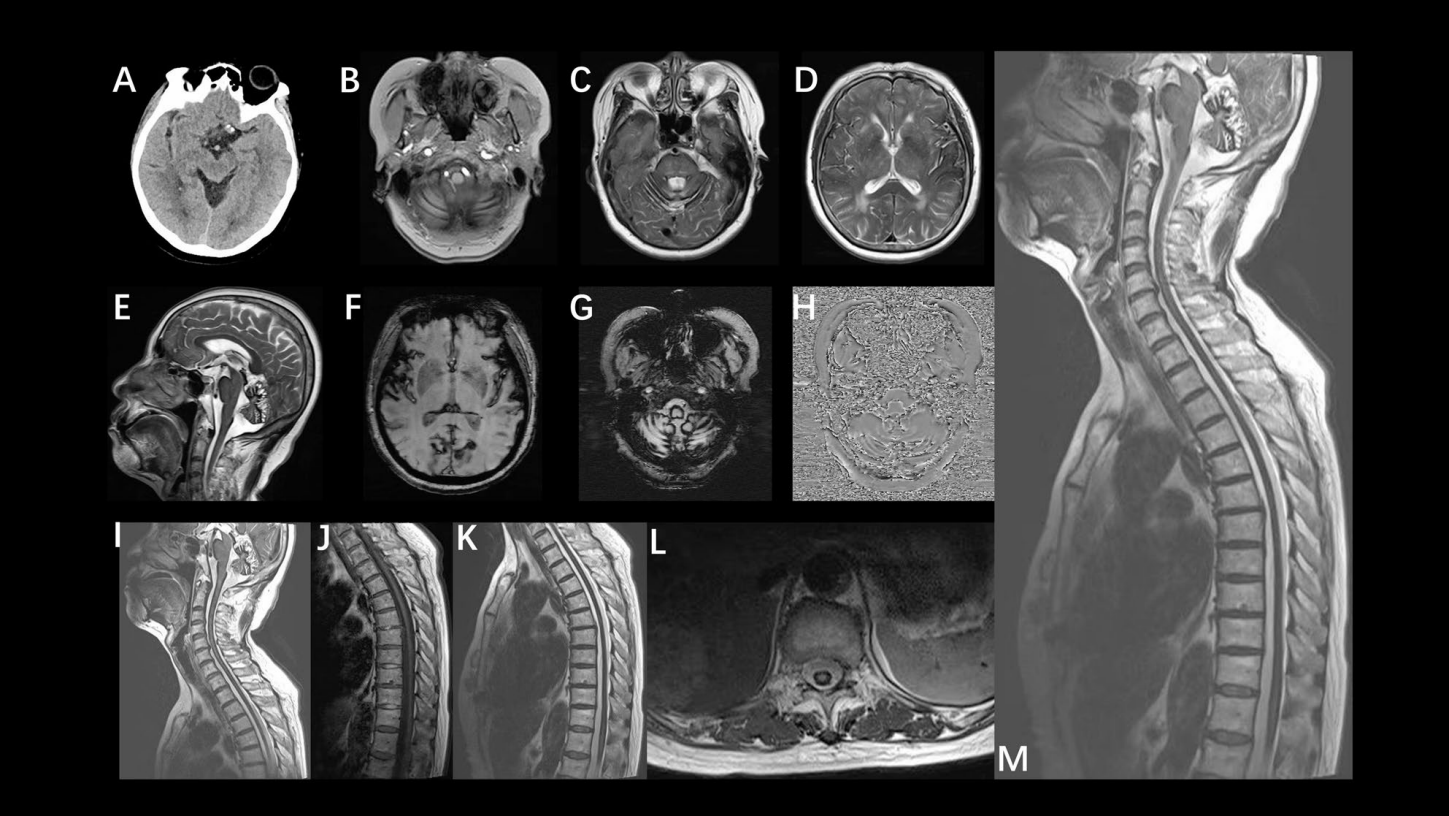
Case 6 A Head CT: the surface of midbrain, pons and along the fourth ventricle was covered with slightly higher linear signal; sagittal and axial Figure B-H head MRI: brainstem and cerebellar atrophy, the surface of brainstem, cerebellum and temporal groove circuit showed slightly higher signal in T1WI sequence, T2WI, DWI and SWI sequence showed uniform low signal coverage on the supratentorial and subtentorial structural surfaces of the cerebellum. I-M MRI of sagittal /cross head and cervicothoracic spine: abnormal signals in the cervical 3–7 and thoracic medullary cord are consistent with the manifestations of SSCNS
